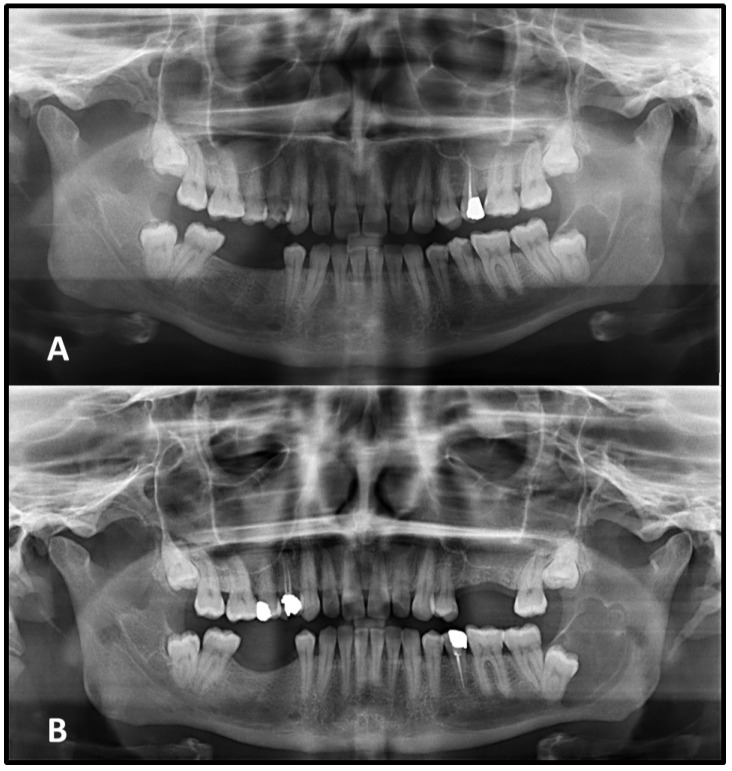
A odontogenic keratocyst with well-defined corticated borders associated with an impacted left mandibular third molar is evident on the initial panoramic radiograph (A). After three years, the lesion had increased in size, causing displacement of the impacted tooth and extending into the inferior alveolar canal (B).
